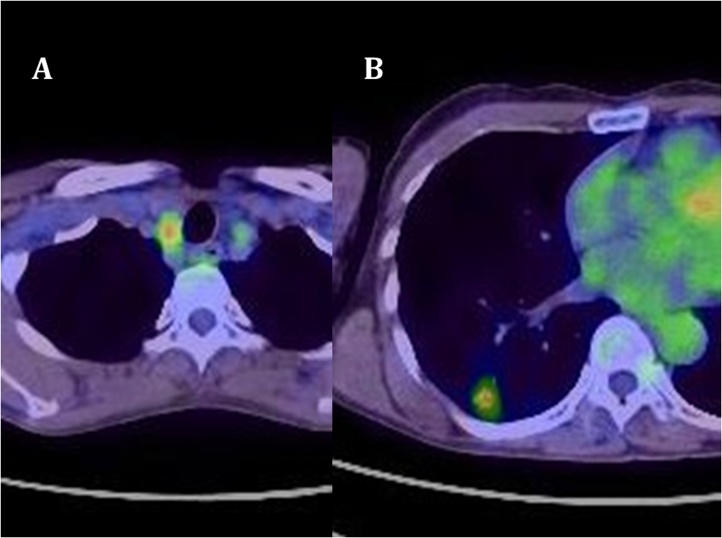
Abnormal uptake areas of fluoro-2 deoxy-D-glucose are seen in the right lobe of the thyroid gland (A) and right lower lung (B) on positron emission tomography – computed tomography imaging.
